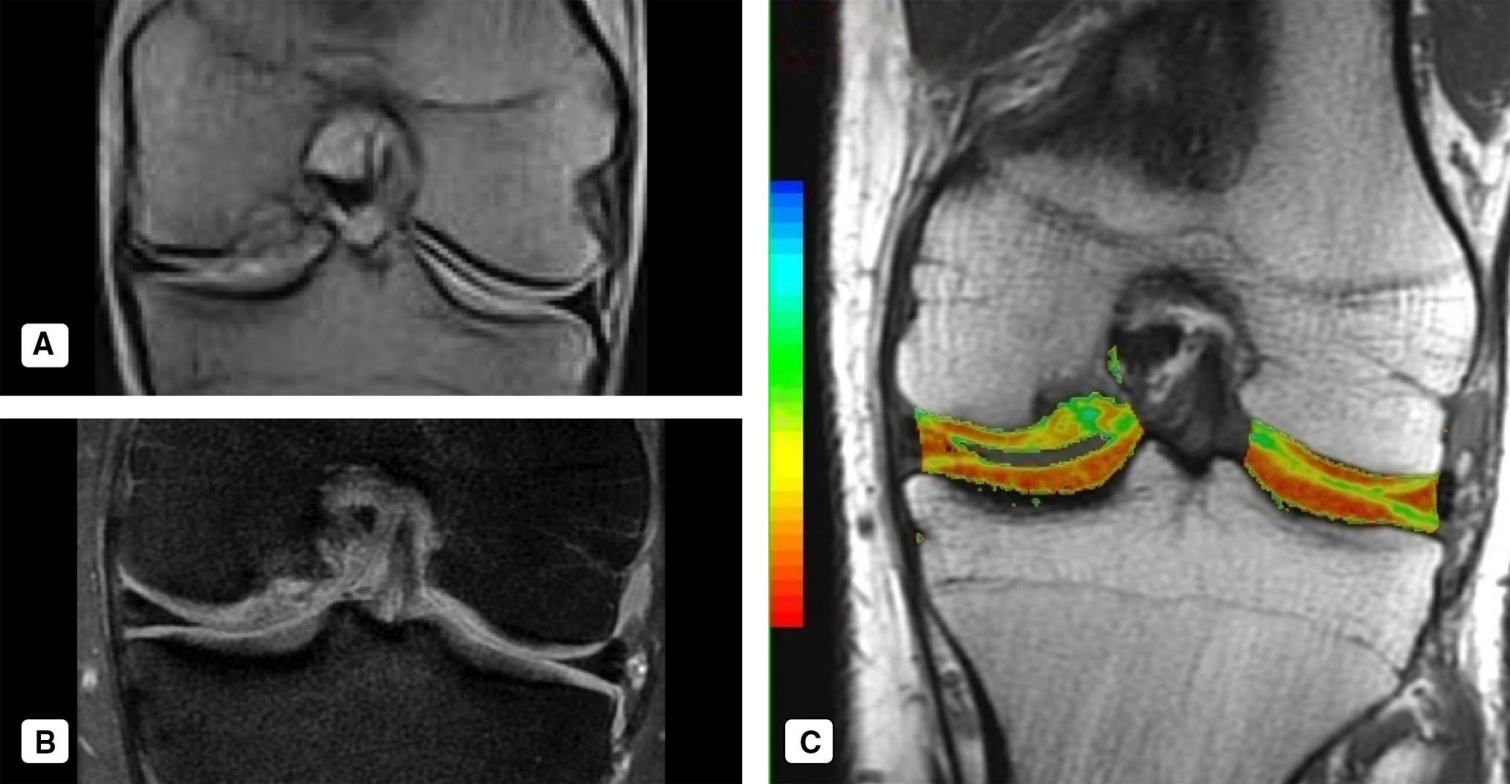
Case imaging. a Pre-Op MRI, b 12 months follow-up MRI, c MOCART Score Evaluation on 12 months follow-up MRI
